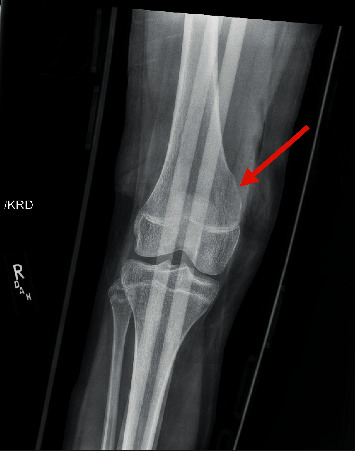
Plain radiograph of the right knee status after surgical excision of the osteochondroma with an immobilizer in place.
